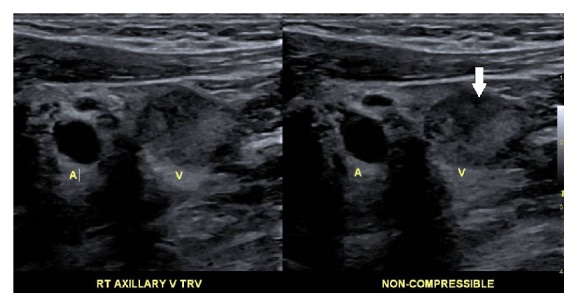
Doppler ultrasound showing noncompressibility of the axillary vein (arrow) due to thrombus.
